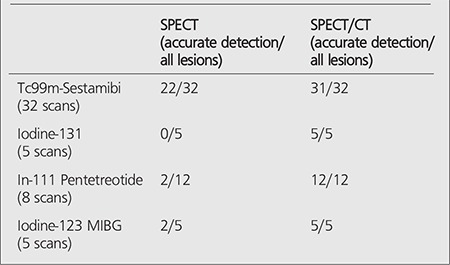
SPECT alone compared with SPECT/CT for accurate localizationand/or characterization of endocrine and neuroendocrine tumors
